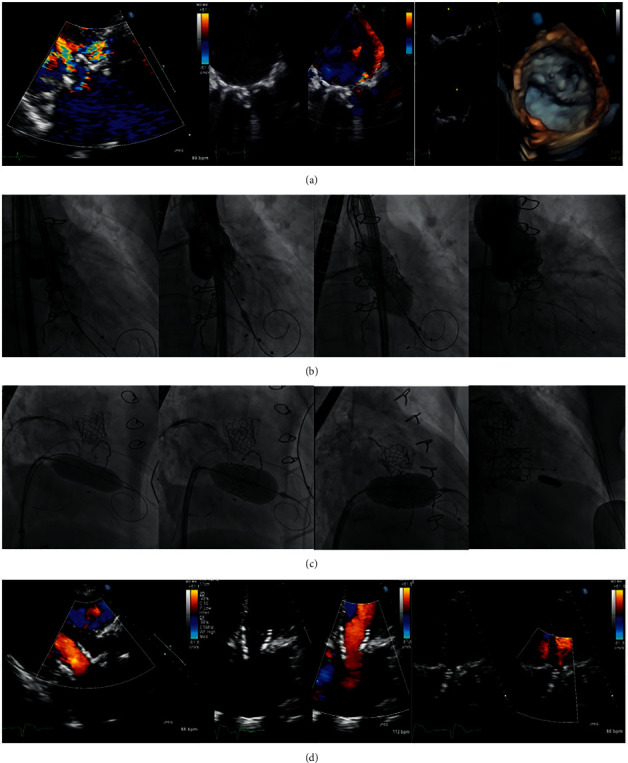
Transcatheter mitral valve in a degenerated Mosaic® ultra™ (Medtronic) valve (TMViV) with a concomitant transcatheter aortic valve implantation (TAVI). A 51-year-old male patient had a degenerated 23-mm Mosaic® ULTRA™ bioprosthetic MV (Medtronic) and a degenerated calcific AV with an associated AF. (a) TTE, TEE, and 3D-TEE show a degenerated bioprosthetic MV (severe MS and severe MR) and a calcific degenerated AV (severe AS and severe AR). (b) Fluoroscopy shows a transaortic TAVI of an Edward SAPIEN-3 26 mm valve during rapid pacing. (c) Fluoroscopy shows pre-TMViV balloon dilatation using a CRISTAL, BALT balloon 22 mm over a 0.035-inch/260 extra-stiff Confida™ guidewire. Then, transseptal TMViV of an Edwards SAPIEN-3 23 mm valve with post-TMViV distal valve flaring, followed by transseptal implantation of a wireless pacemaker as the patient developed CHB. (d) TTE: both SAPIEN-3 valves are in mitral and aortic positions with normal flow across both valves. AF: atrial fibrillation, AR: aortic regurgitation, AS: aortic stenosis, AV: aortic valve, CHB: complete heart block, 3D: three-dimensional, MR: mitral regurgitation, MS: mitral stenosis, MV: mitral valve, MVA: mitral valve area, Pg: pressure gradient, TAVI: transcatheter aortic valve implantation, TMViV: transcatheter mitral valve-in-valve, TEE: transesophageal echocardiography, and TTE: transthoracic echocardiography.
